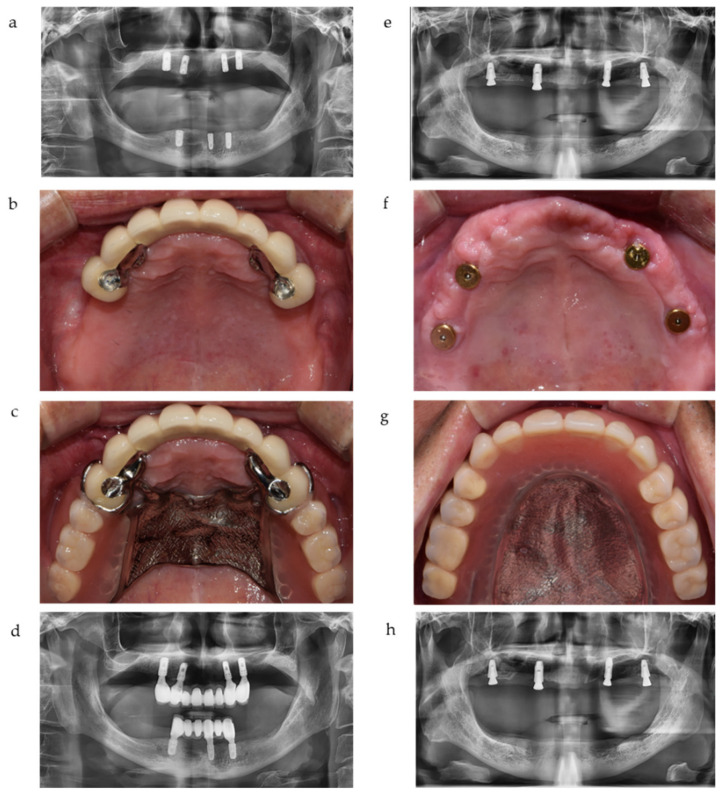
Representative cases of implant-crown-retained removable partial dentures (IC-RPD) and implant overdentures (IOD) in this study. (a) Implants were placed in anterior positions due to anatomical limitations. (b) Intraoral view of IC-RPD case. (c) Delivery of IC-RPD. (d) Panoramic radiograph of IC-RPD case. (e) Four implants were placed with lateral sinus floor elevation and bone augmentation. (f) Intraoral view of IOD case. (g) Delivery of IOD. (h) Panoramic radiograph of IOD case.
